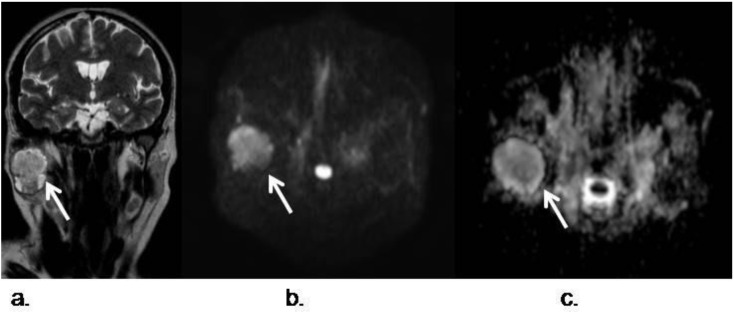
Pleomorphic adenoma of the right parotid gland; (a) Coronal TSE T2-weighted image shows a right intraparotid well defined lesion (white arrow); (b) Transverse DWI acquired with a *b* value of 800 s/mm^2^ demosntrates hyperintensity of the lesion which is also hypointense in the corresponding ADC map (c), indicating restriction of free water diffusion. The mean ADC value was 1.6 × 10^−3^ mm^2^/s. In this case, DWI favors pleomorphic and helps to distinguish it from a Warthin tumor.
